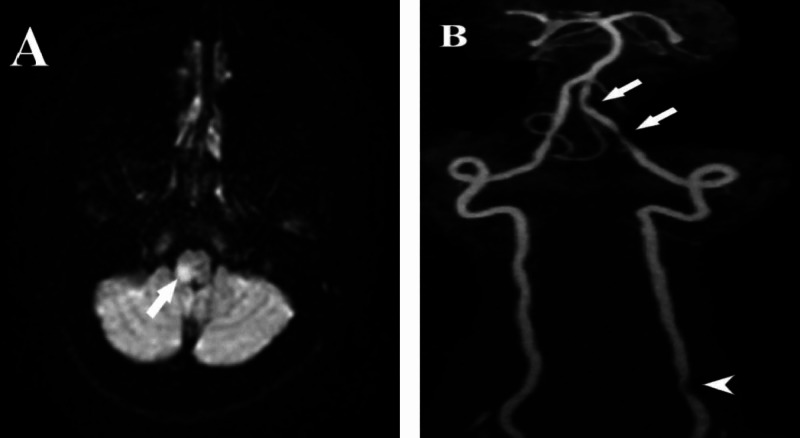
MRI and MRA findings – case 2 (A) Brain MRI, DWI sequence showing restricted diffusion within the right lateral medulla (arrow). (B) Brain MRA showing multiple focal stenotic lesions in the right vertebral artery (V4) segment (arrows), and (V1) segment (head arrow) MRI: magnetic resonance imaging; DWI: diffusion-weighted imaging; MRA: magnetic resonance angiography
